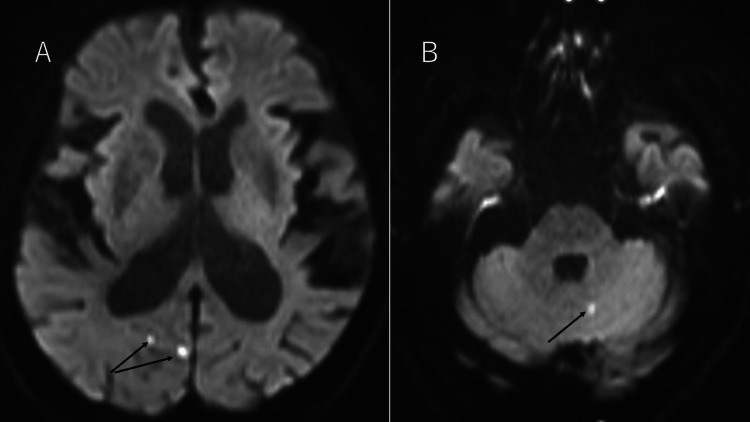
(A) Diffusion-weighted images in brain magnetic resonance imaging (MRI). (B) Diffusion-weighted images in MRI in the cerebellar hemisphere. In (A) and (B), arrows show the portion of acute cerebral infarction.
